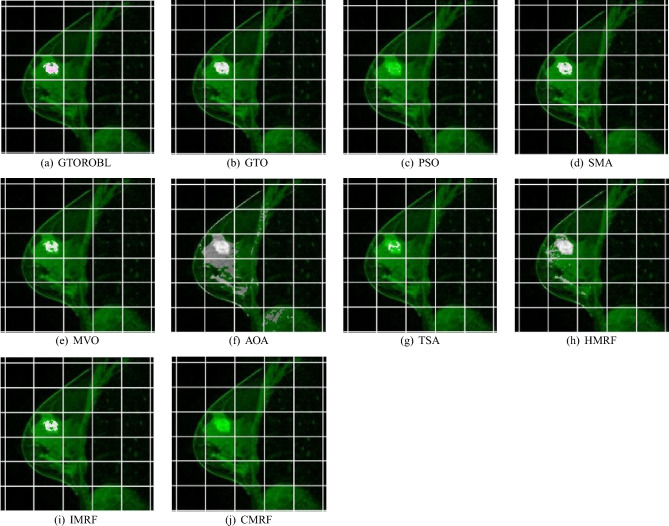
Localized lesions (bright colored spot) in MR images for patient-1 (a) GTORBL, (b) GTO, (c) PSO, (d) SMA, (e) MVO, (f) AOA, (g) TSA, (h) HMRF (i) IMRF, and (j) CMRF.
